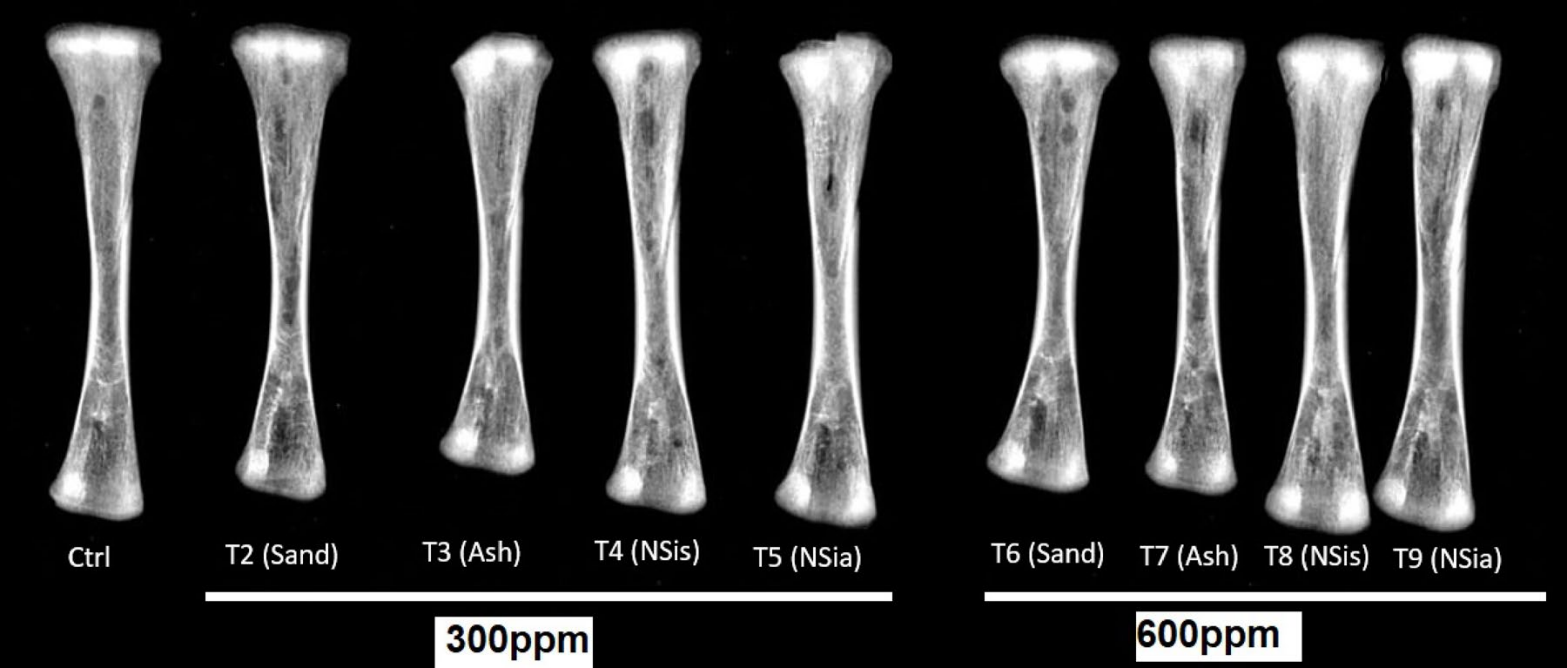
X-Ray image was taken on tibia bone.
